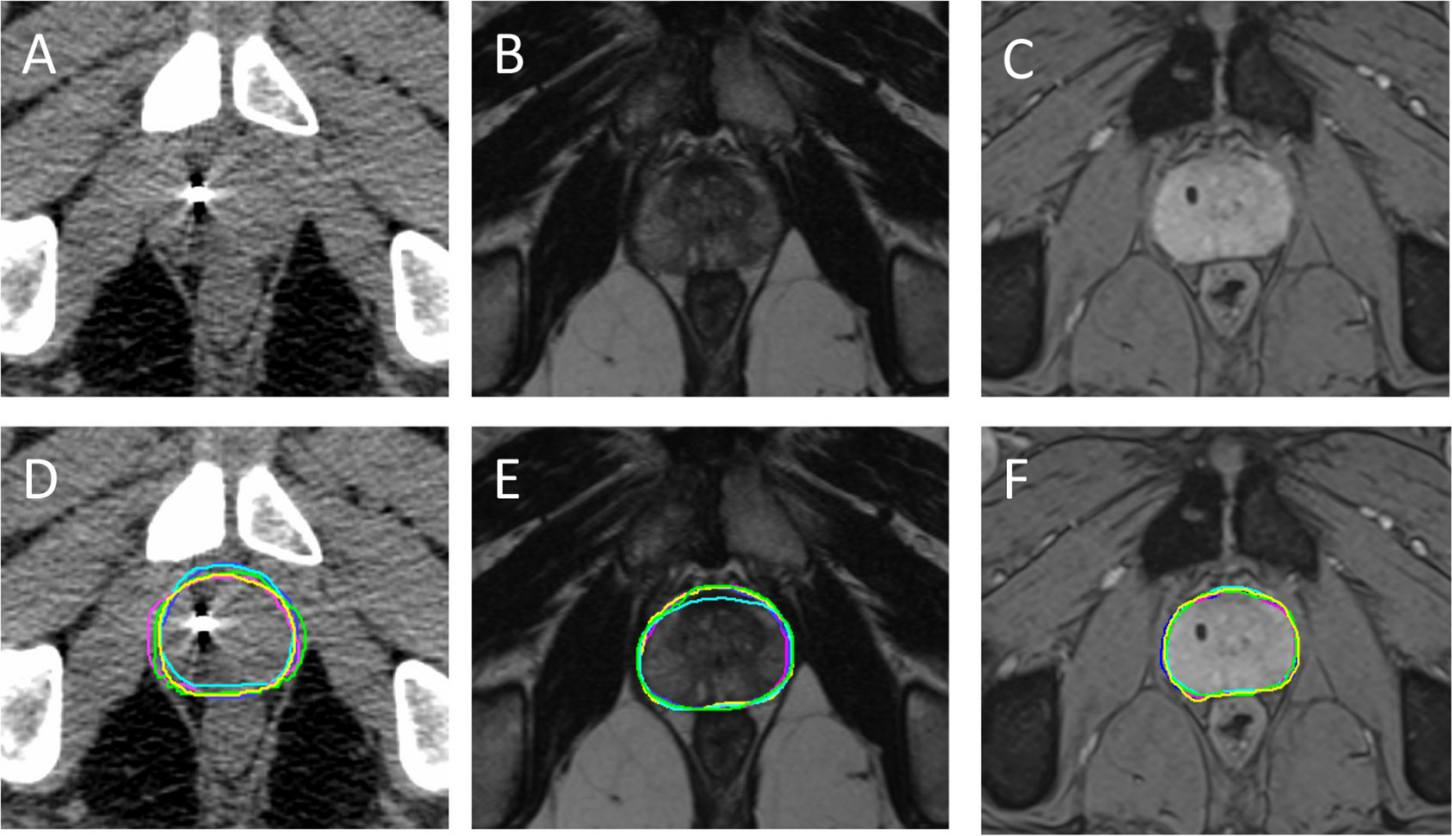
A–C are examples of CT, *T*_2_W and *T*_2_*W imaging at corresponding levels for the same patient, without contours. D–F demonstrate the same imaging with superimposed radiographer contours. Reproduced from published abstract format with permission.^13^
